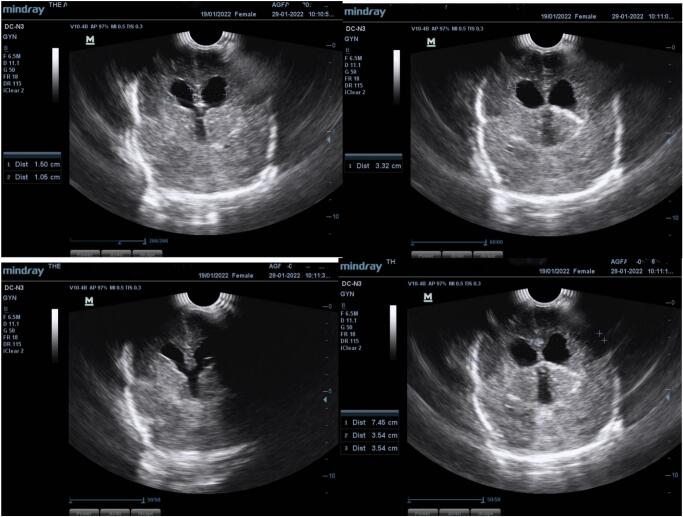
Cranial ultrasound scan done 1 week post meningoencephalocele repair: The lateral, third and fourth ventricles are dilated, the dilatation has significantly increased since the previous scan. There are subtle bilateral cortical hypoechoic areas affecting the fronto-temporal region. The paraventricular regions are normal. There is diffuse lack of sulci and gyri.
